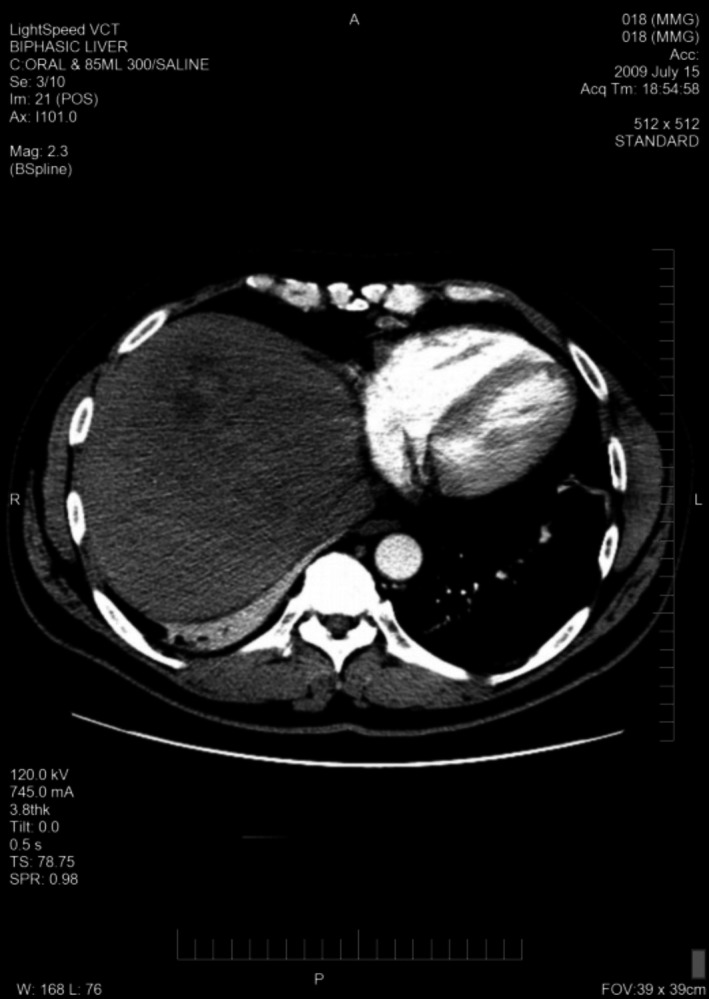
Patient 1 with Hepatocellular Carcinoma (HCC)—Baseline CT images—arterial phase.
